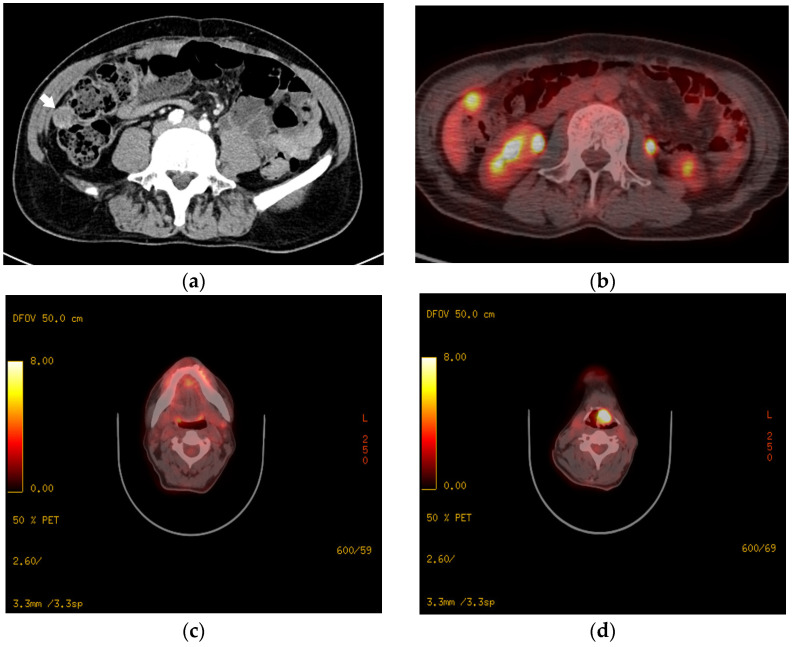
(a) Computed tomography (CT) of the abdomen and pelvis revealed an approximately 20 mm lesion in the right lower quadrant (white arrow). On positron emission tomography-CT, hypermetabolic lesions were seen in (b) the subhepatic area, (c) the left submandibular region, and (d) the epiglottis.
